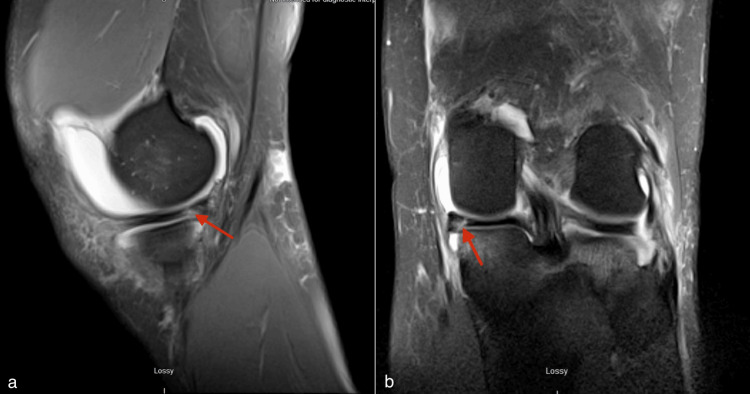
Preoperative MRI image of the left knee a) T2 sagittal magnetic resonance imagining of the preoperative left knee demonstrating the vertical medial meniscus tear (red arrow). b) T2 coronal magnetic resonance imagining of the preoperative left knee with a vertical medial meniscus tear (red arrow).
